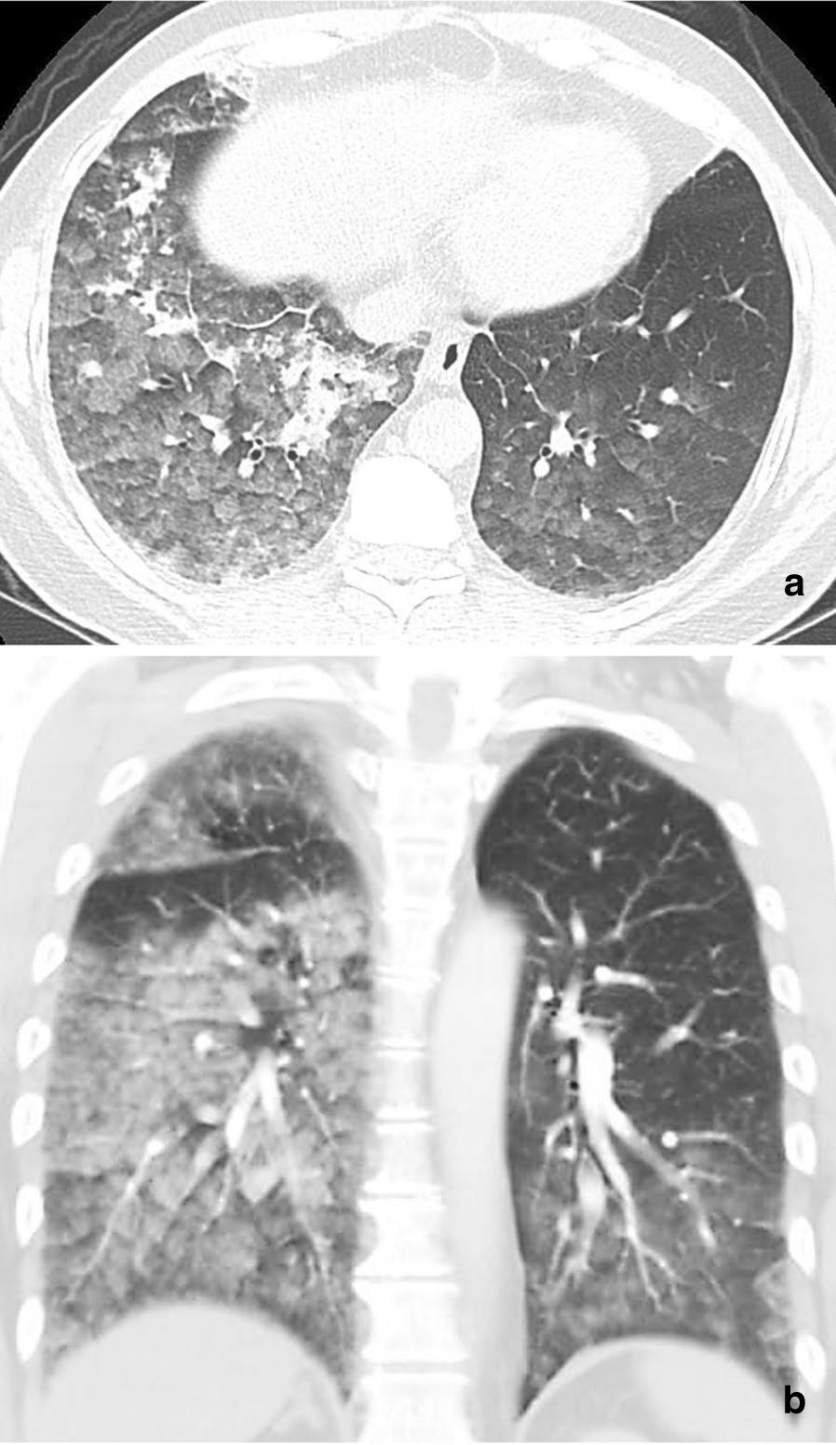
Non-infectious mimickers of indeterminate COVID-19. A 63-year-old man with diffuse alveolar hemorrhage secondary to thrombolytic therapy for myocardial infarction. a, b axial and coronal reformatted HRCT images show asymmetric bilateral patchy areas of GGO with small areas of consolidation
